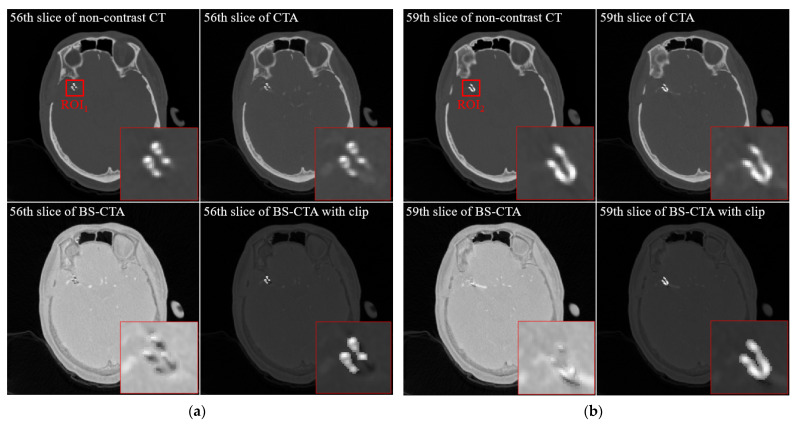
Examples of the non-contrast CT (left upper), the CTA (right upper), the BS-CTA (left bottom), and the proposed BS-CTA (right bottom); (a) 56th slice and (b) 59th slice.
